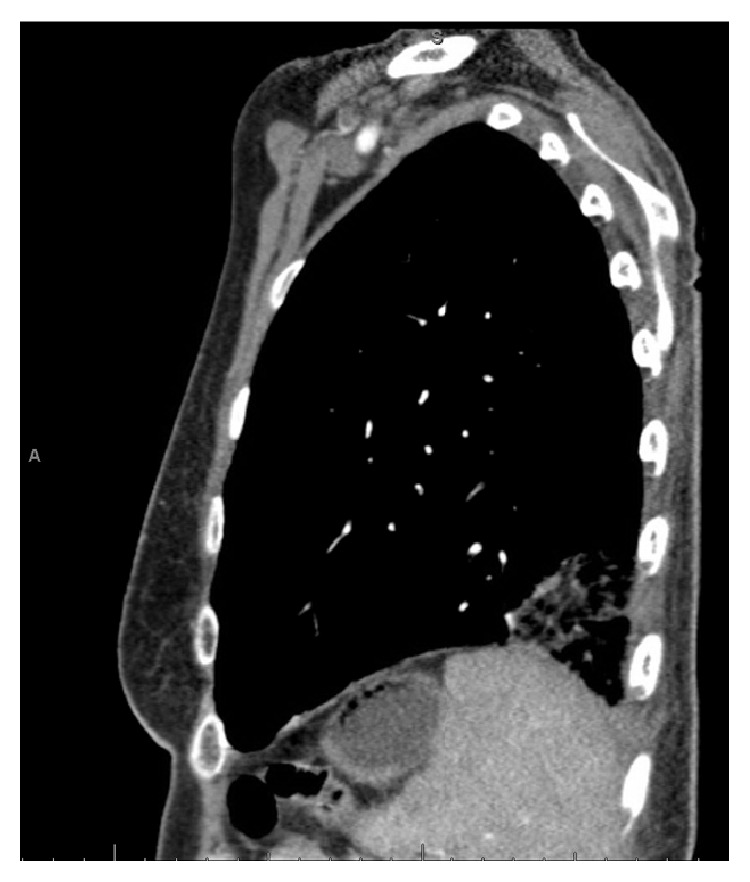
Chest CT scan of case 2 showing pulmonary embolism.
